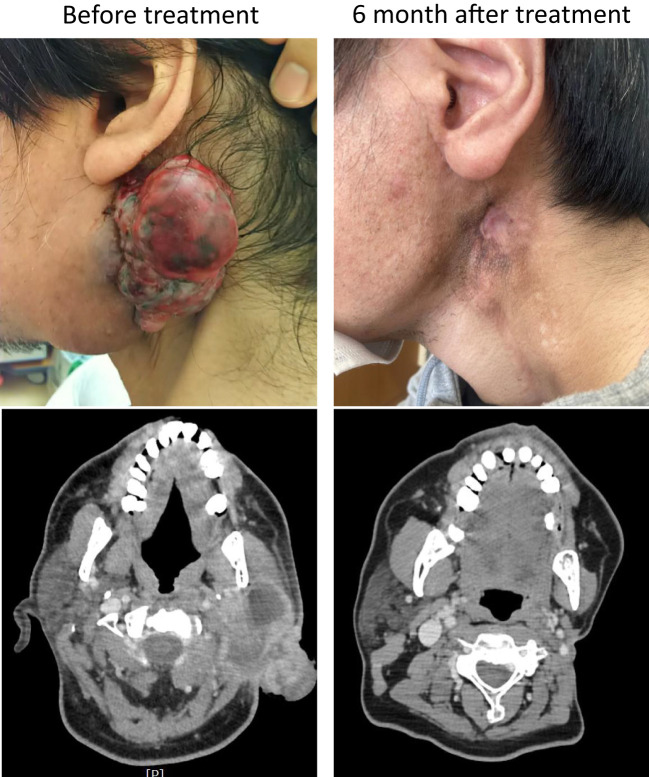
Gross and computed tomography (CT) images. The upper panels show the gross findings of the local recurrence site before and after treatment, and the lower panels show the CT images of the same site before and after treatment.
